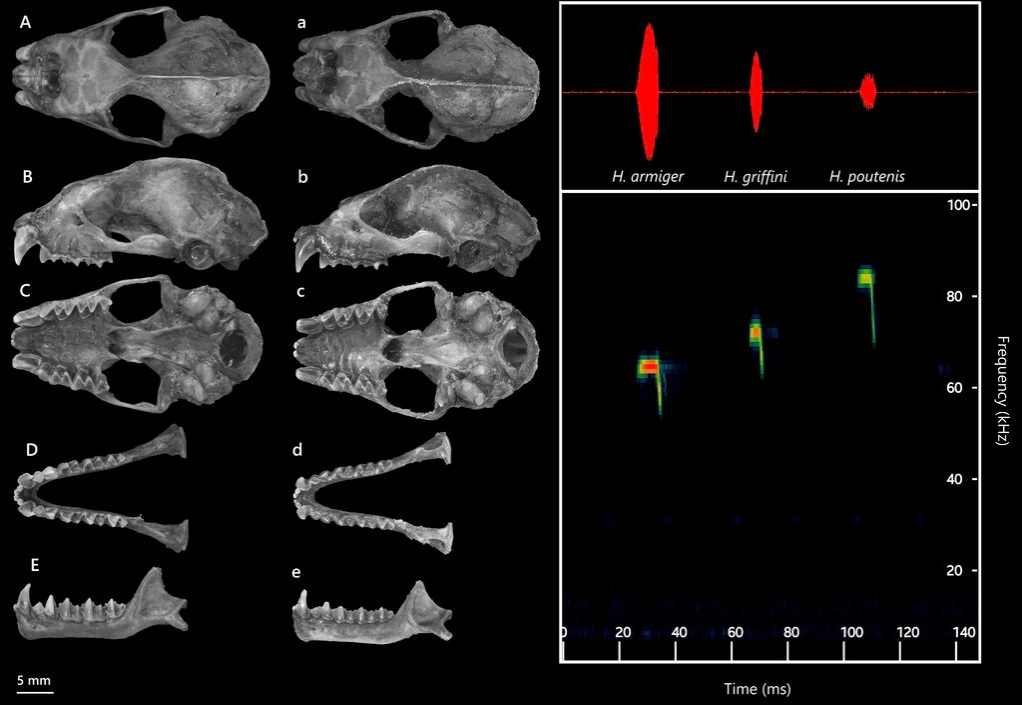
(Left) Dorsal (A, a), lateral (B, b), ventral (C, c) views of the cranium and dorsal (D, d), lateral (E, e) views of the mandible, comparing two closely-related species: *H*.* armiger *and *H*.* griffini*; (Right) Ultrasonic echolocation calls of Hipposideridae bats.
